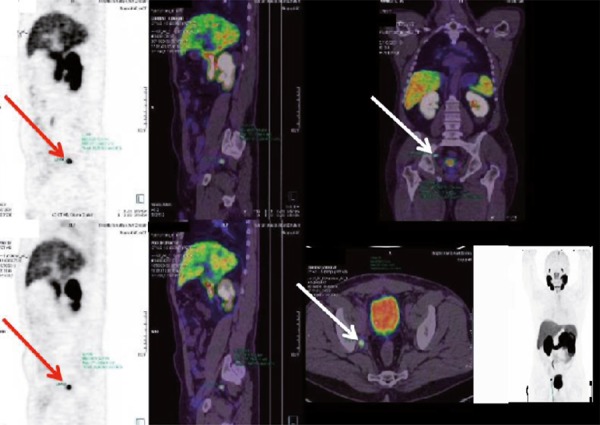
68Ga-PSMA PET/CT (fusion images) revealed an increased uptake in gallium Ga 68 (68Ga) - labeled PSMA (SUV=45.8) in suspected LN with 1.0cm in the right obturator fossa.
